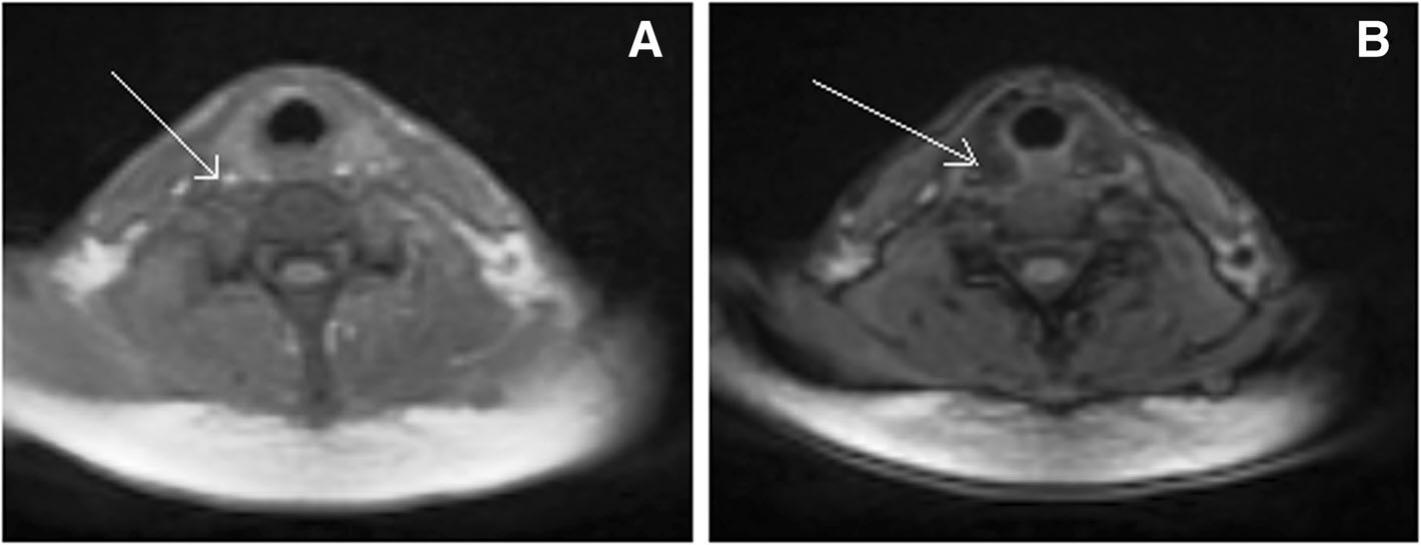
Cervical MRI for patient 1 with In-*Phase* sequence (IP) (a) and O*ut*-Of-*Phase* sequence (OOP) (b) showing drop of signal intensities. Cervical MRI for patient 1 shows lipid-rich thyroid gland. In-Phase sequence (IP) (a) demonstrates an intermediate signal (arrow). Out-of-phase sequence (b), thyroid gland shows a reduction of signal intensity (arrow), indicating that the gland is most likely infiltrated by lipids. This signal intensity difference is related to difference between water and fat protons resonance frequencies
